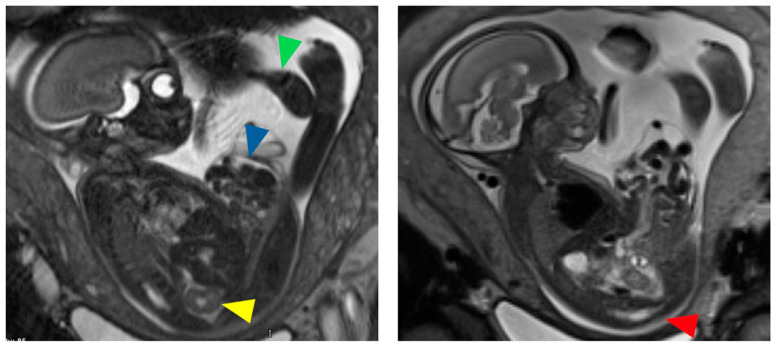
Fetal MRI images of a female fetus at 24 weeks gestation with characteristic findings of cloacal exstrophy including large omphalocele (blue arrow), absent urinary bladder, right pelvic kidney with absent left kidney (yellow arrow), left club foot (green arrow), and low spinal cord termination with truncated sacrum and coccyx (red arrow).
